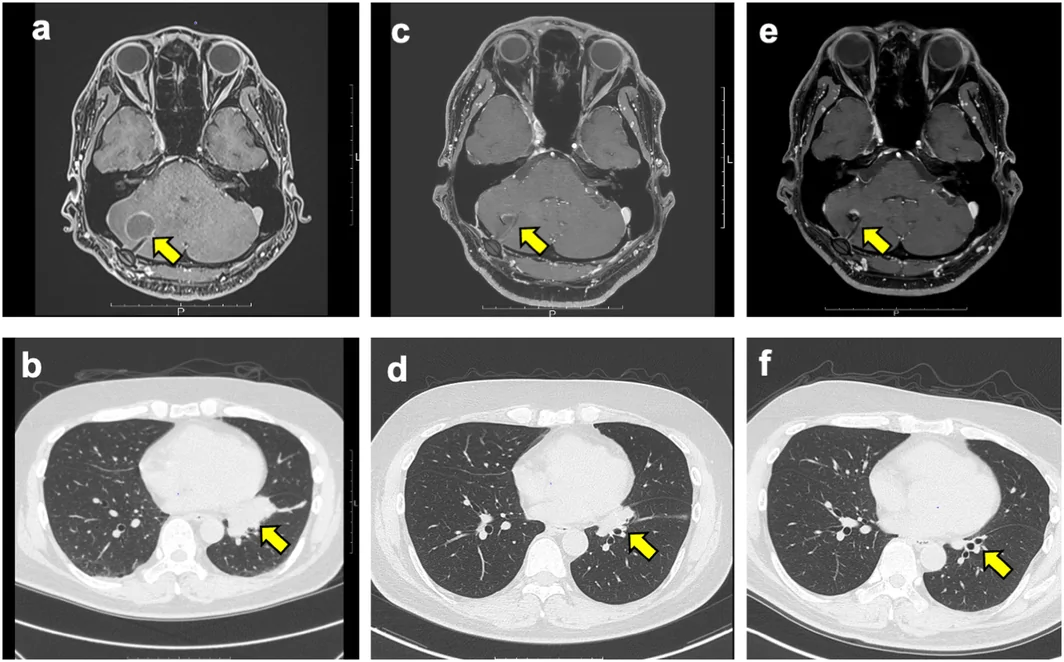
Radiological findings of the present case. (a, c, e) Brain MRI performed before initiation of lorlatinib (a), at 2 months (c), and at 14 months (e), demonstrating marked regression of brain metastases and sustained intracranial disease control. (b, d, f) Representative CT images obtained before lorlatinib initiation (b), at 3 months after treatment initiation (d), and at 17 months during continued therapy (f). Baseline chest CT revealed a tumour lesion in the left lower lobe (b). Follow‐up CT at 3 months showed marked tumour reduction (d), and sustained disease control without progression was maintained at 17 months (f).
